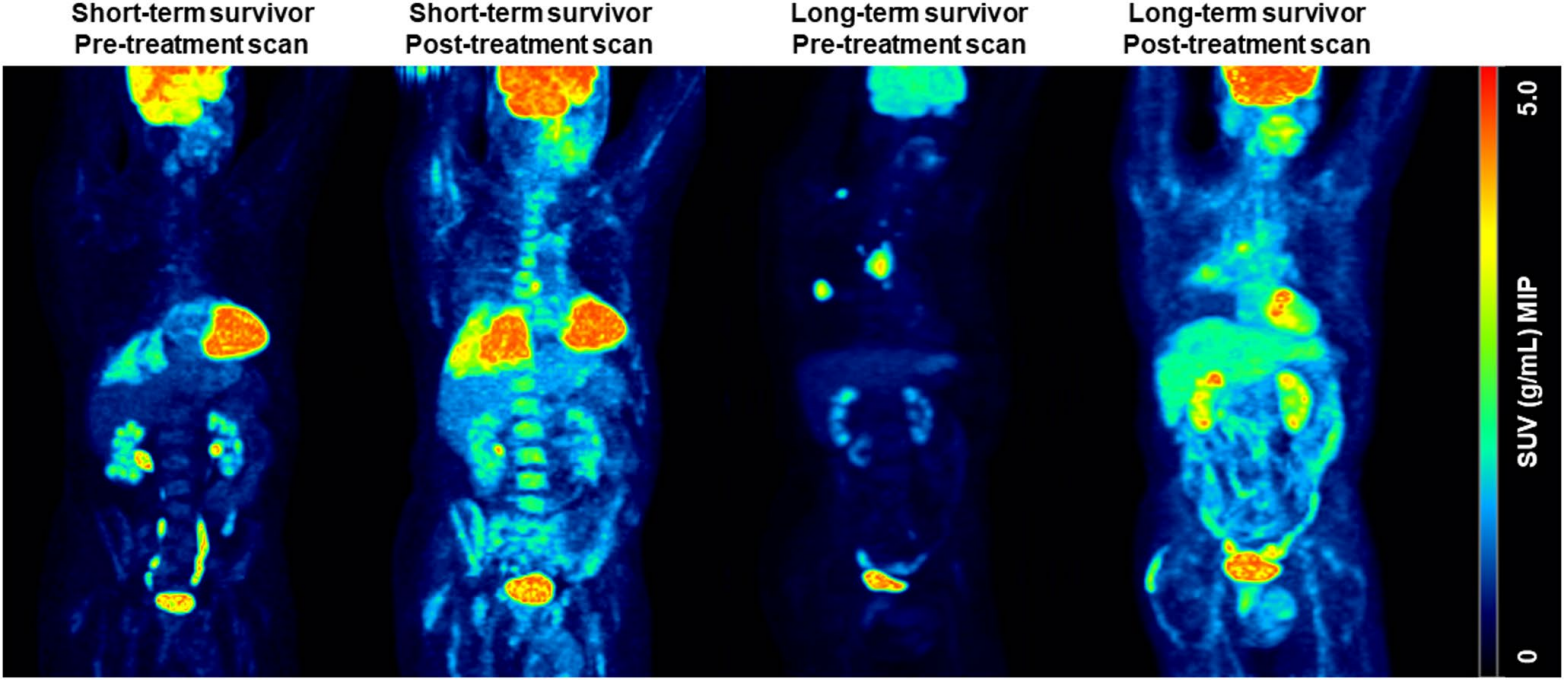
Representative maximum intensity projection (MIP) standardised uptake value (SUV) [^18^F]FDG PET images of NSCLC patients before and after treatment. From left to right: Short-term survivor [^18^F]FDG PET image pre-treatment; Short-term survivor [^18^F]FDG PET image post-treatment; Long-term survivor [^18^F]FDG PET image pre-treatment; and Long-term survivor [^18^F]FDG PET image post-treatment. Note significantly visible bone uptake in the pre- and post-treatment [^18^F]FDG PET image of the short-term survivor versus long-term survivor
